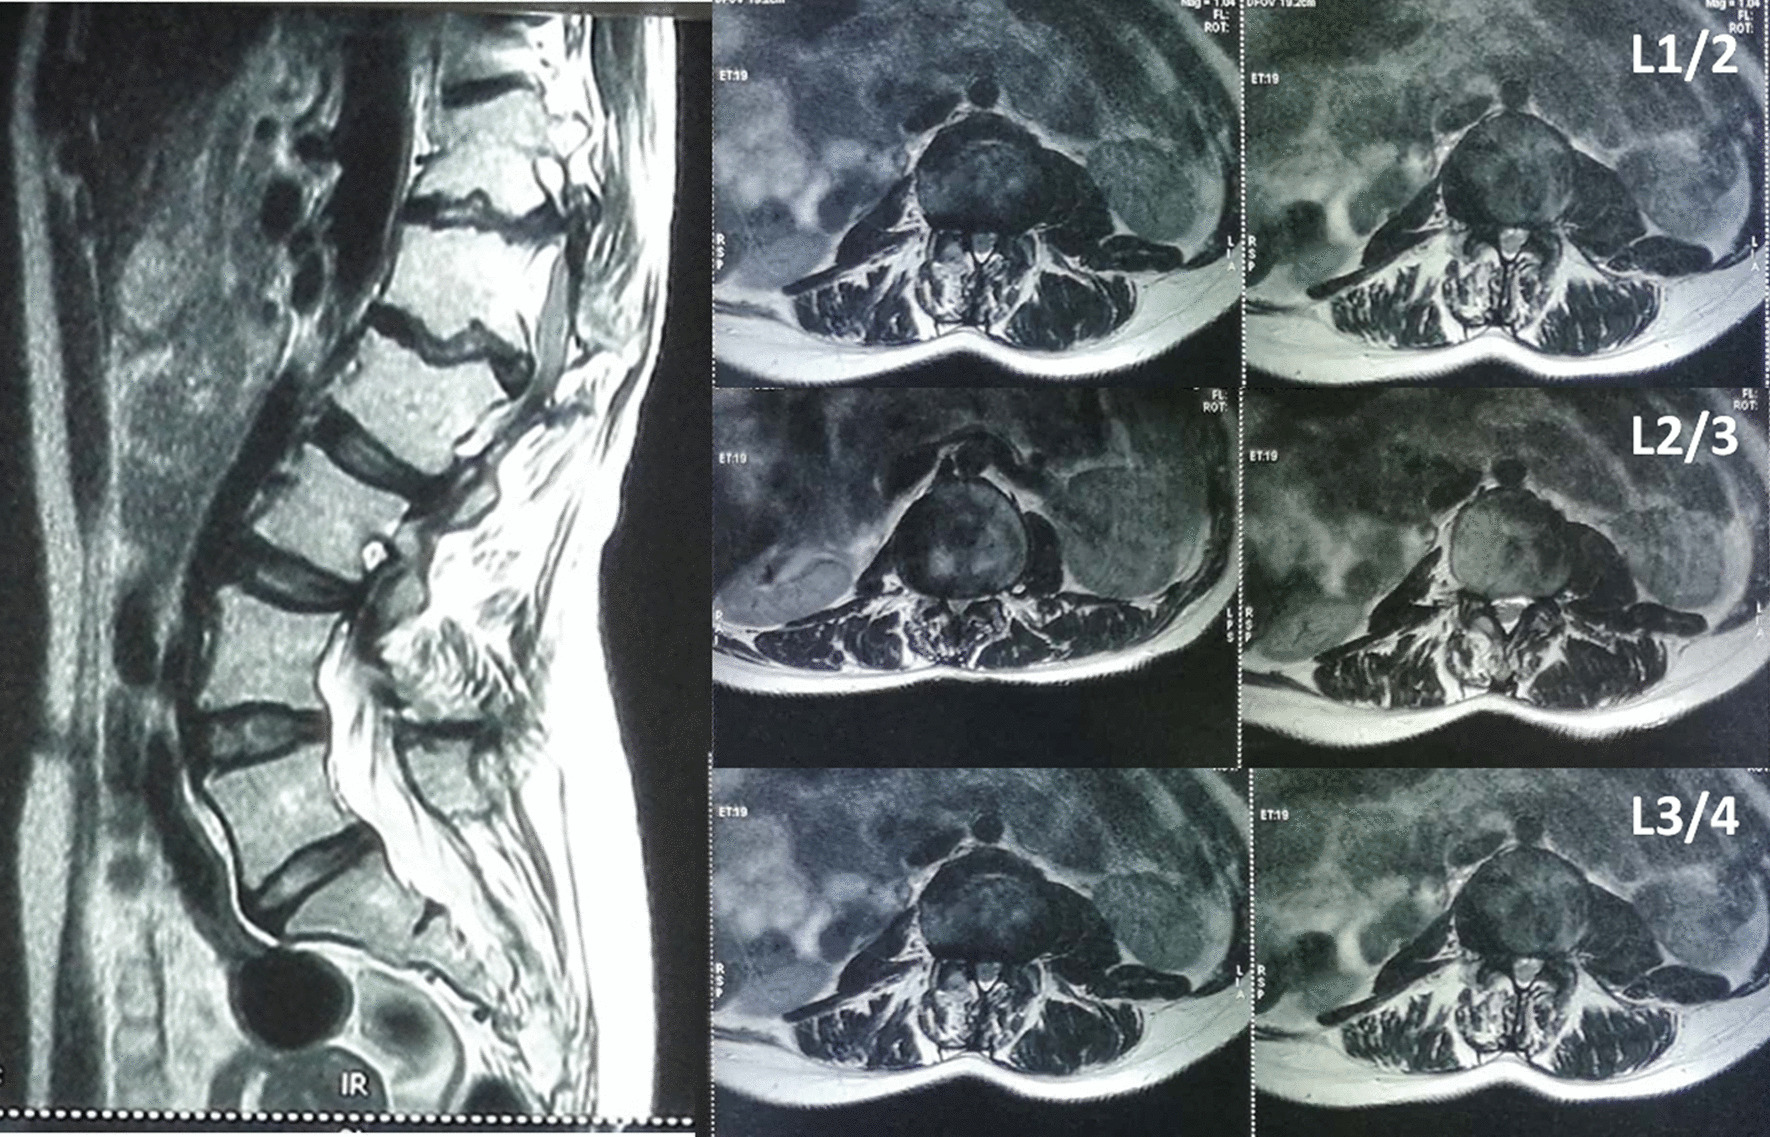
Sagittal and axial MRI of the lumbar spine showed multi-level severe upper lumbar spinal stenosis (L1/2,L2/3,L3/4) caused by posterior osteophytes and short pedicle
